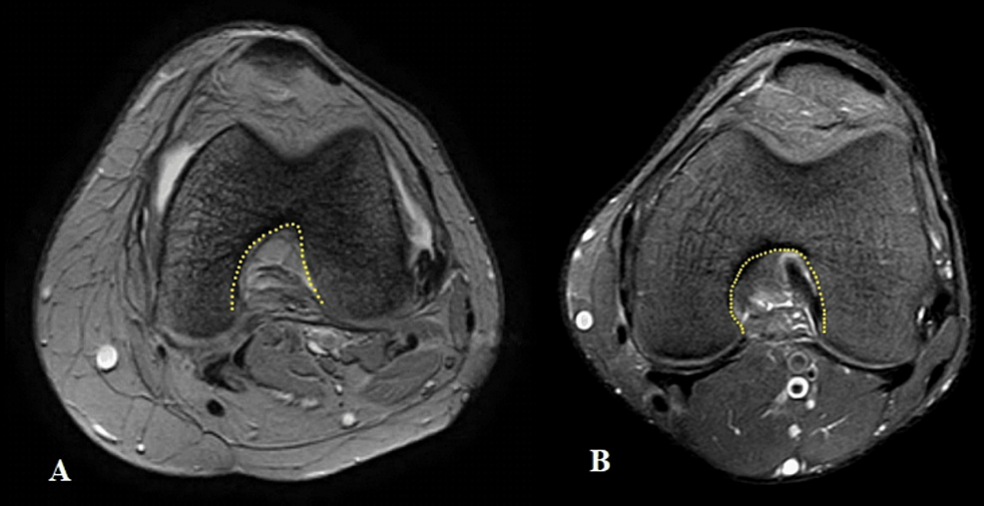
Axial MRI images: proton density (PD) weighted fast spin echo (FSE) sequence depicting the "A"-shaped notch (A) and the "U"-shaped notch (B), respectively.
